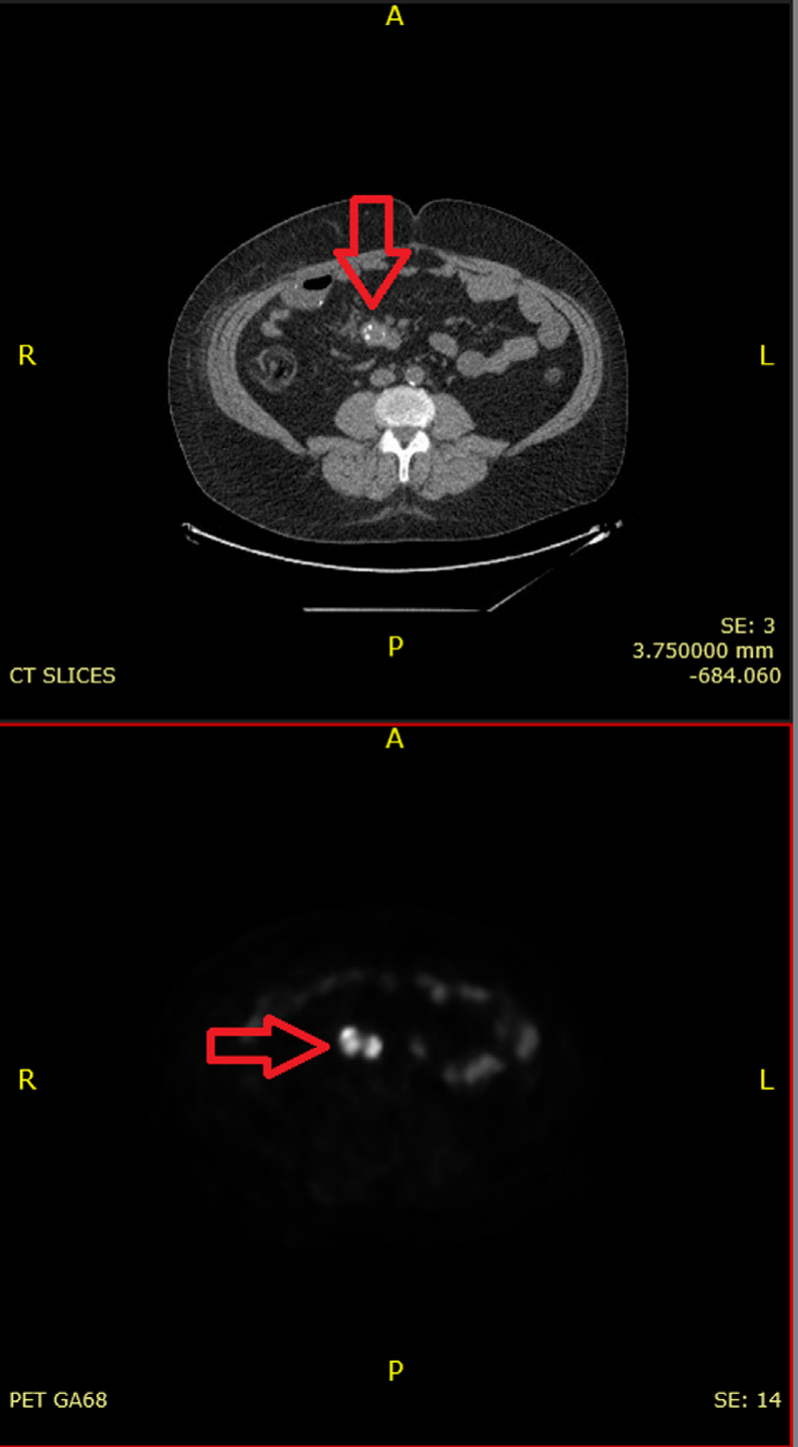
Gallium-68 Positron Emission Tomography/Computed Tomography Scan Gallium-68 positron emission tomography/computed tomography scan showing intense pathologic uptake in the mesenteric mass (arrow).
